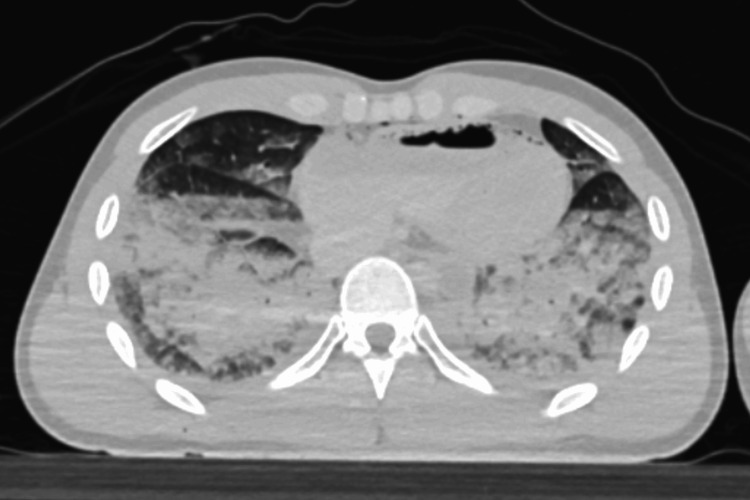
Postmortem CT imaging of the lungs Axial non-contrast postmortem CT image shows diffuse ground-glass opacities and fluid accumulation in both lungs, consistent with pulmonary edema.
